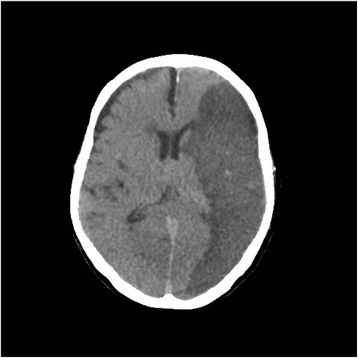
Computed tomography showed the large cerebral infarction on day 29 after admission
